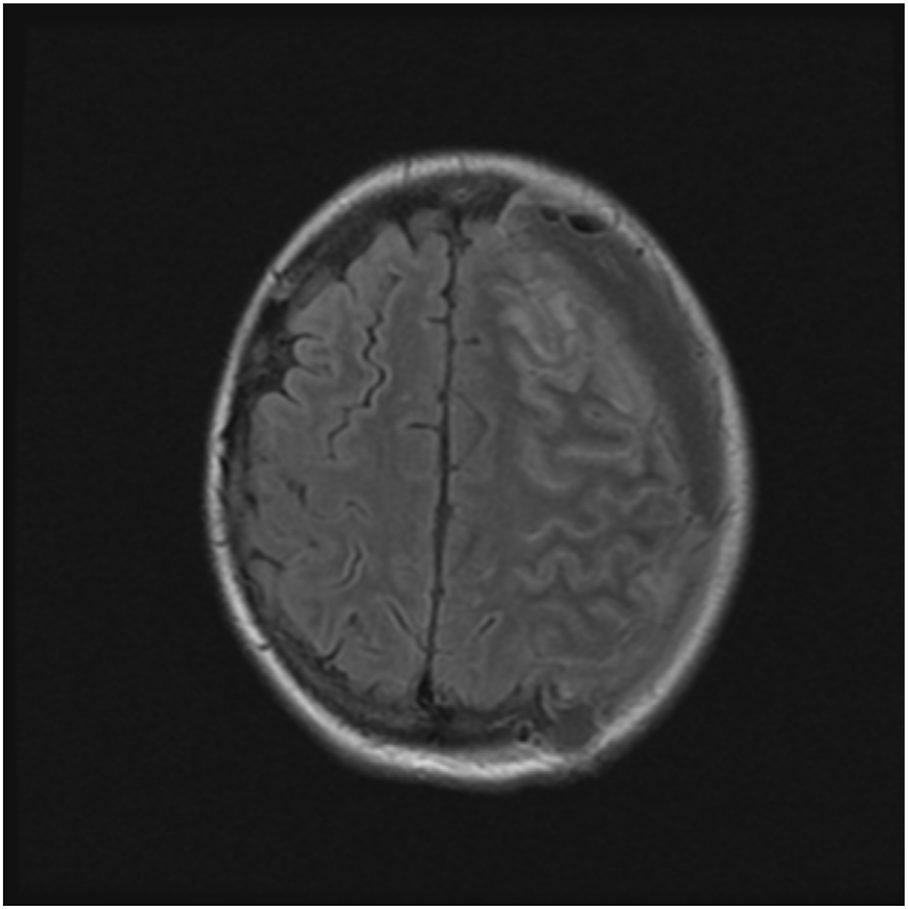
MRI Brain with and without contrast T2/FLAIR: Hyperintensity involving left frontal gyrus concerning for focal cerebritis of the left anterior frontal lobe.
